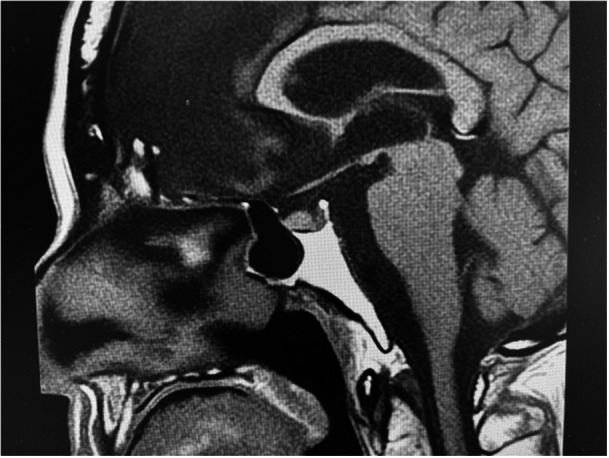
Magnetic resonance imaging (MRI) revealed loss of signal from the posterior pituitary gland.
